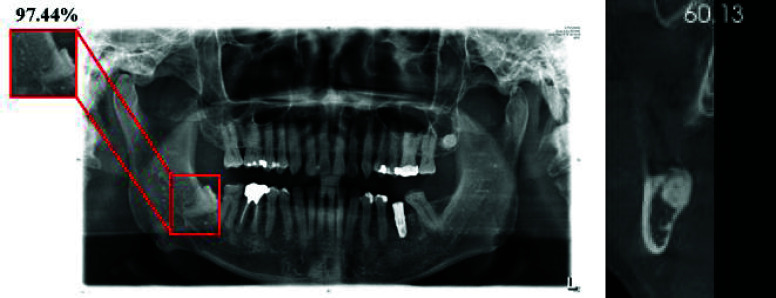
The validation results with DPR and the CT image for supplementary assessment.
